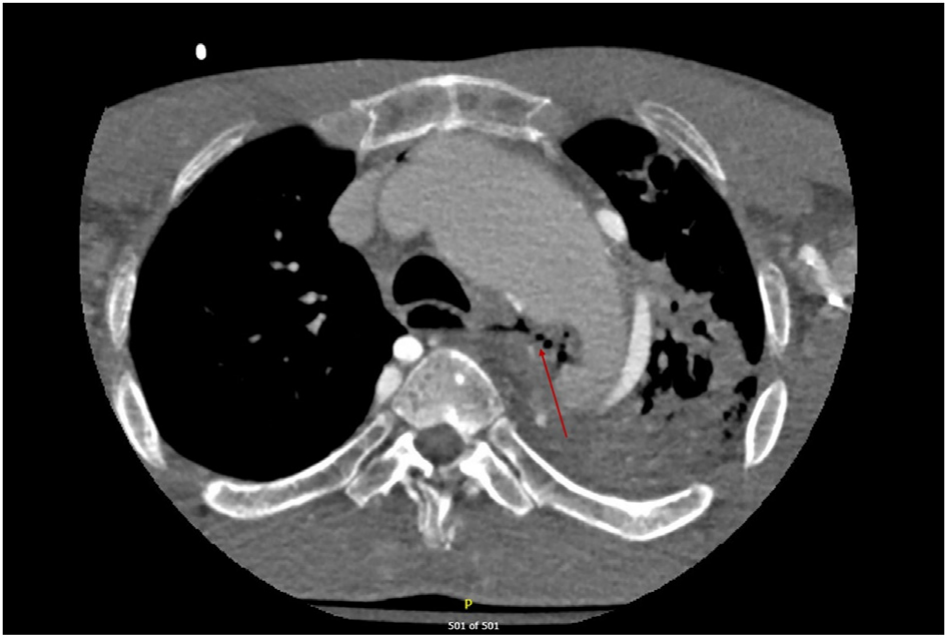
Computed tomography scan of the patient’s chest shortly after presentation to the emergency department. Red arrow shows gas tracking from esophagus to aorta concerning for aortoesophageal fistula. Left upper lobe consolidation visible at bottom right of image.
